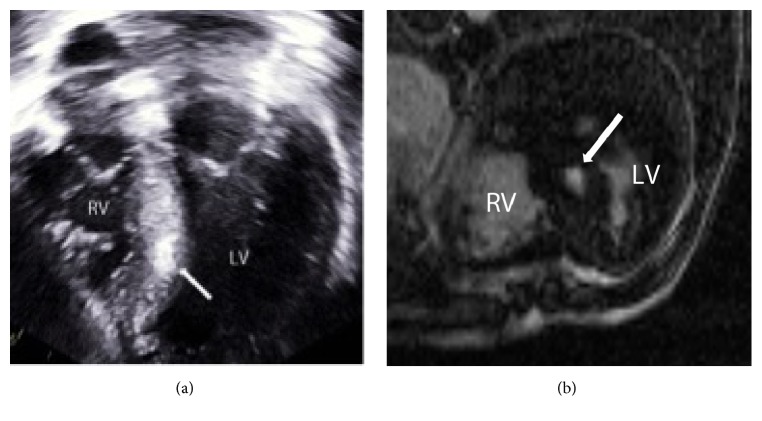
Sample images from echocardiography (a) and cMRI (b) performed on the same patient demonstrating positive findings (white arrows) of hyperechogenicity of the septal myocardium on echocardiography and LGE in the same location at cMRI (RV: right ventricle and LV: left ventricle).
